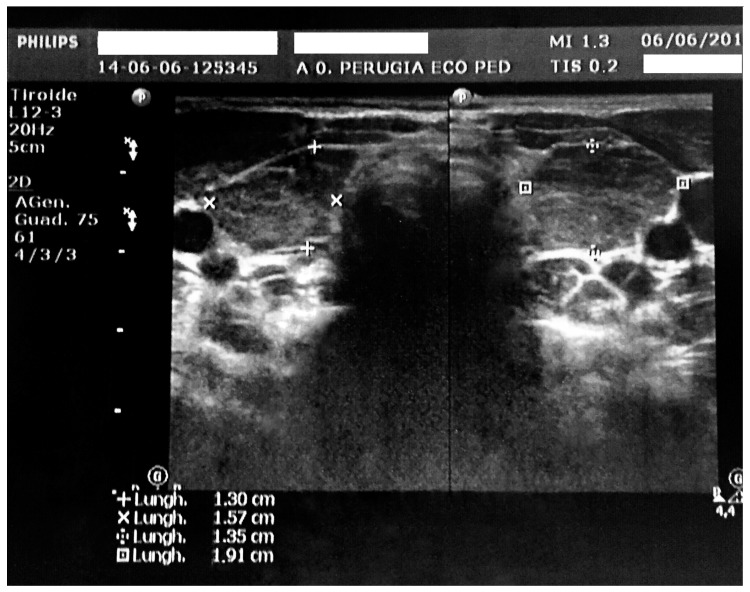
Thyroid ultrasound with evidence of increased gland dimensions and a non-homogeneous echotexture aspect.
